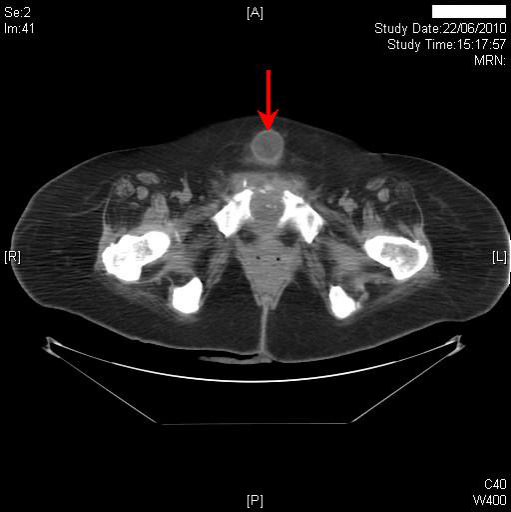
Computed tomography of abdomen revealed the suprapubic catheter lying outside the bladder. The balloon (red arrow) and tip of the catheter were located in the subcutaneous adipose layer superficial to the lower part of the rectus sheath.
